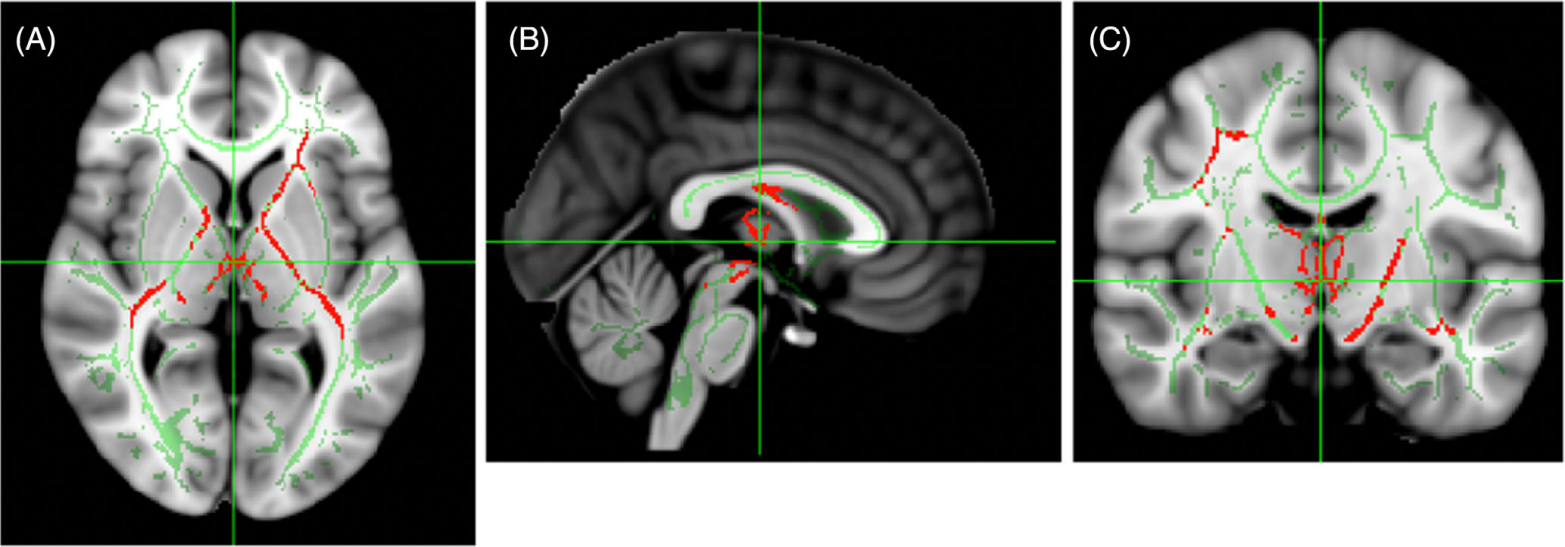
Functional magnetic resonance imaging from an individual with argininosuccinic aciduria (ASA) showed involvement of basal ganglia. (A–C) Decreased fraction of anisotropy in the internal capsule, fornix and white‐matter tracts in the vicinity of basal ganglia in an individual with ASA with (A) axial, (B) sagittal and (C) coronal sections.
